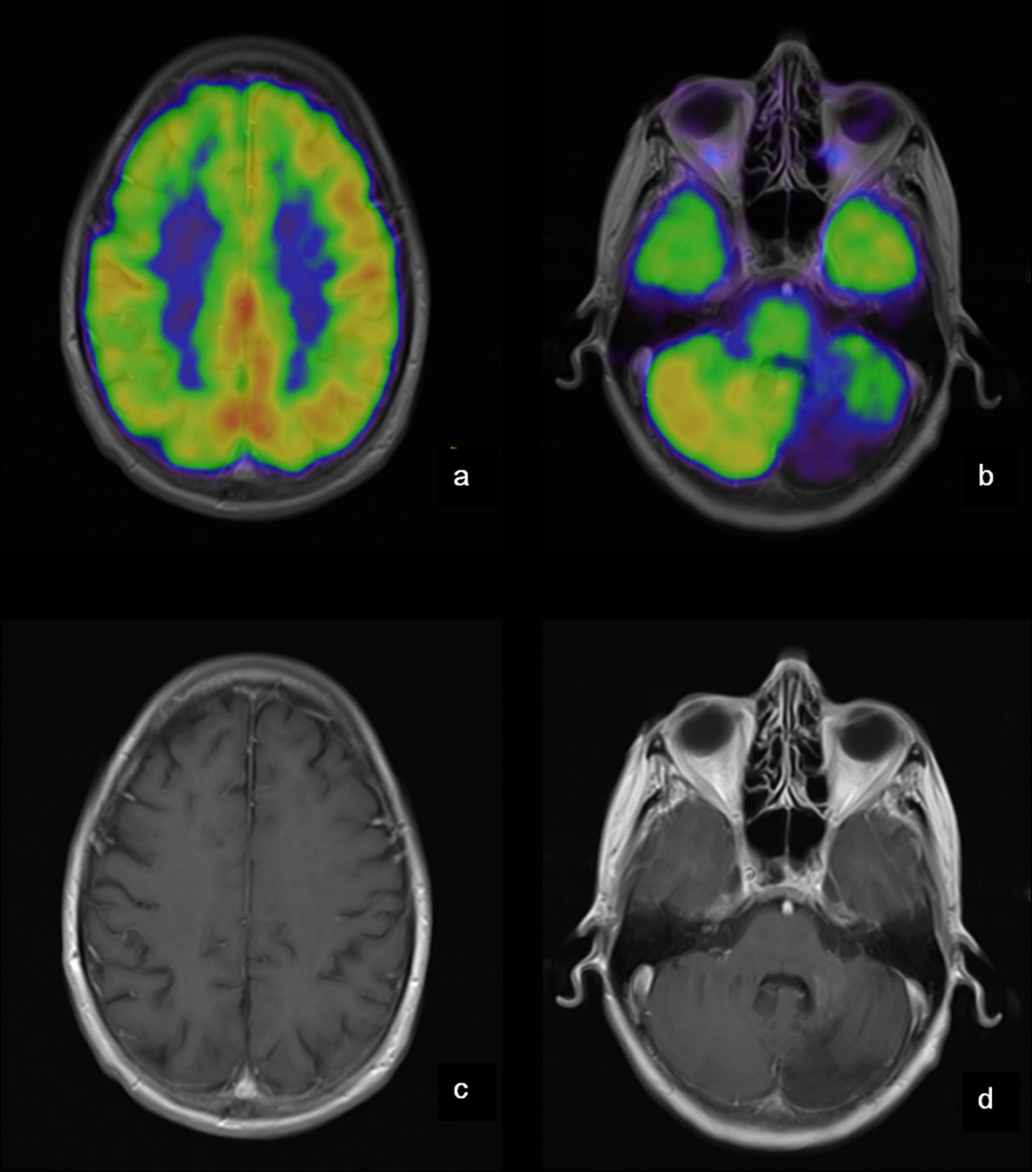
PET and MRI of the brain of patient no 25. Patient no. 25 suffered from a cerebellar hemorrhage and developed a hypometabolic area corresponding to tissue loss in the left cerebellar hemisphere (b + d) in addition to a cerebello-cortical diaschisis (a + b). a: Cortex (MRI fusioned with PET)–decreased activity in the right hemisphere. b: Cerebellum (MRI fusioned with PET)–decreased activity in the left cerebellar hemisphere. c: Cortex (MRI)–no structural changes. d: Cerebellum (MRI)–sequelae after hemorrhage.
